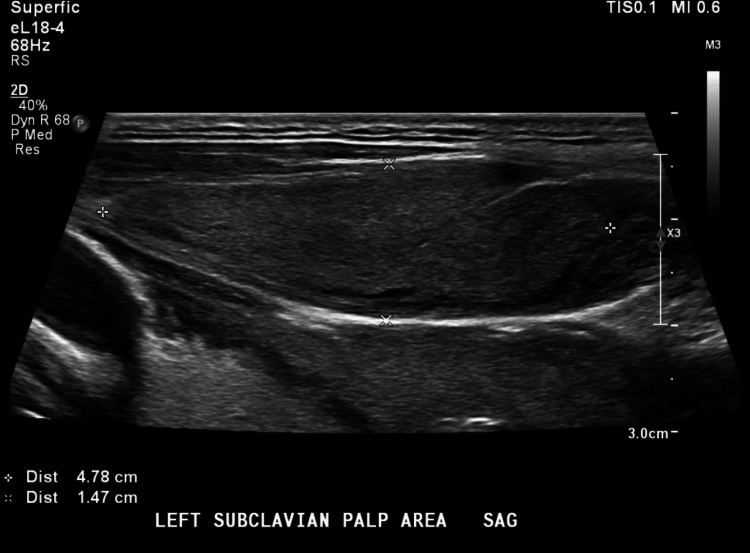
Ultrasound of the neck showing a left supraclavicular abscess.
